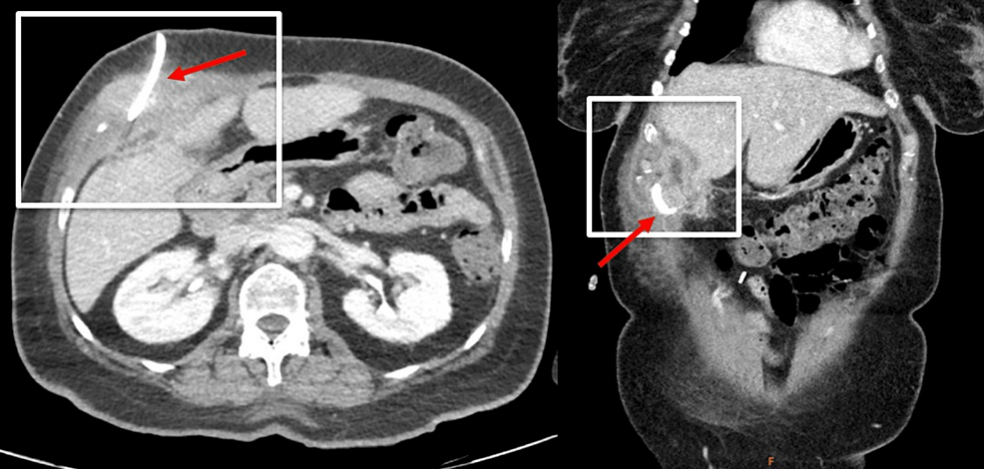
Post-drain placement follow-up CT showing interval decrease of the abdominal wall abscess Arrow: catheter in the center of the abscess
